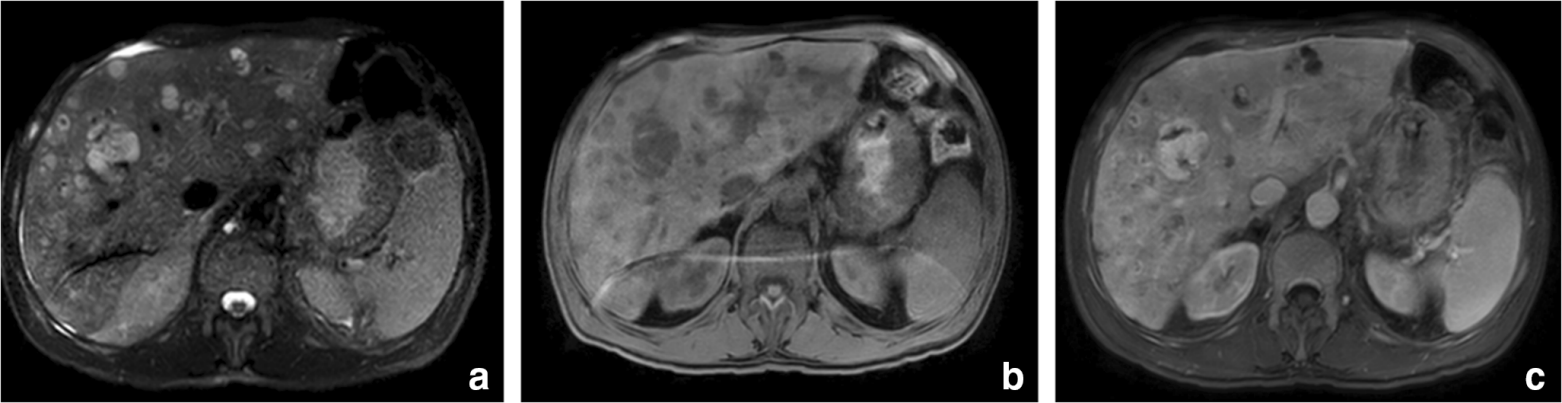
MRI: multiple focal liver lesions appearing hyperintense on T2 (a) and hypointense on T1 (b). After contrast media injection the lesions show fill in appearing hyperintense on venous phase (c)
